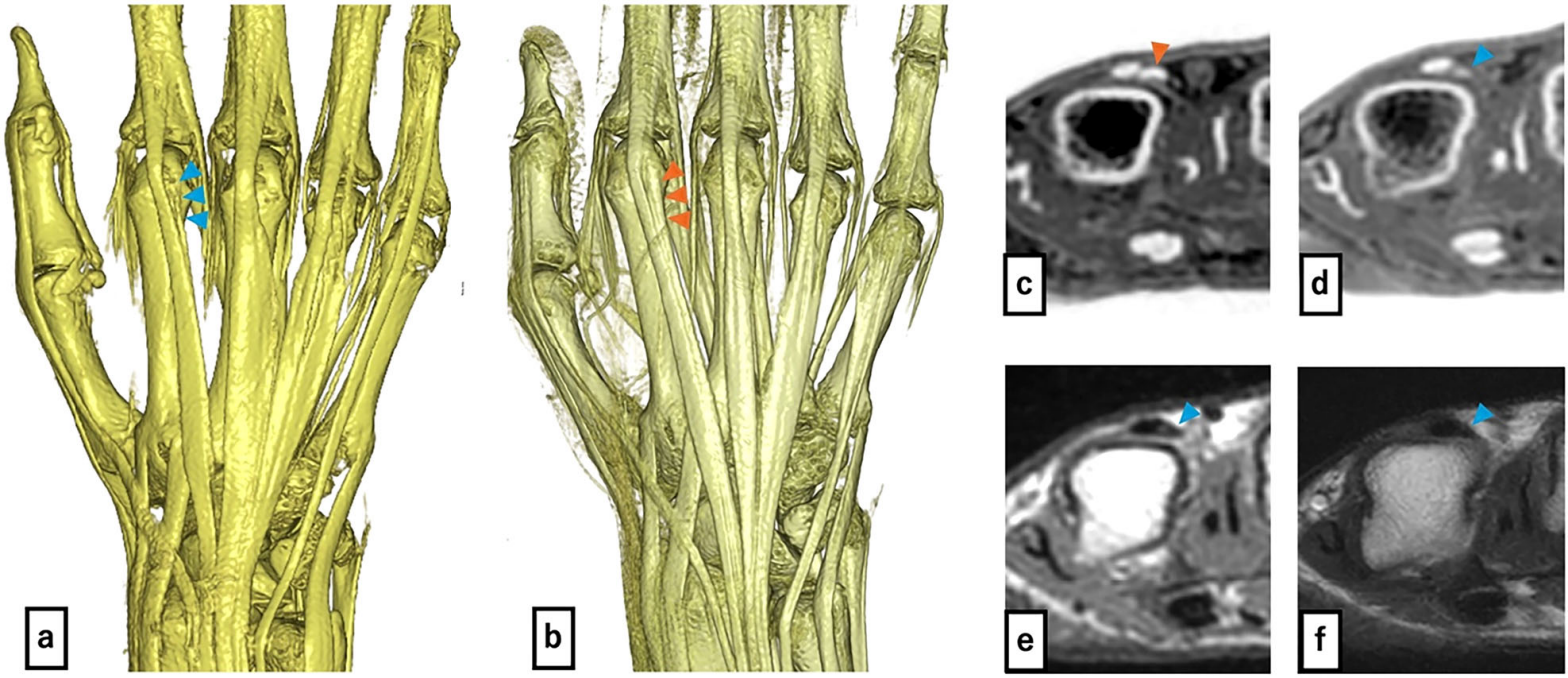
Volume-rendered images from the FRACTURE sequence: (a) case with EIP hypoplasia, (b) case with normal EIP. Comparison shows the hypoplastic EIP in a (blue and orange triangles: EIP). Axial FRACTURE sequence: (c) case with EIP hypoplasia, (d) case with normal EIP. e Axial PD-VISTA sequence of a case with EIP hypoplasia, (f) axial T2-weighted imaging sequence of a case with EIP hypoplasia. The EIP tendon in c appears hypoplastic when compared to d. The EIP cannot be clearly identified in e and f, making assessment of hypoplasia not feasible
